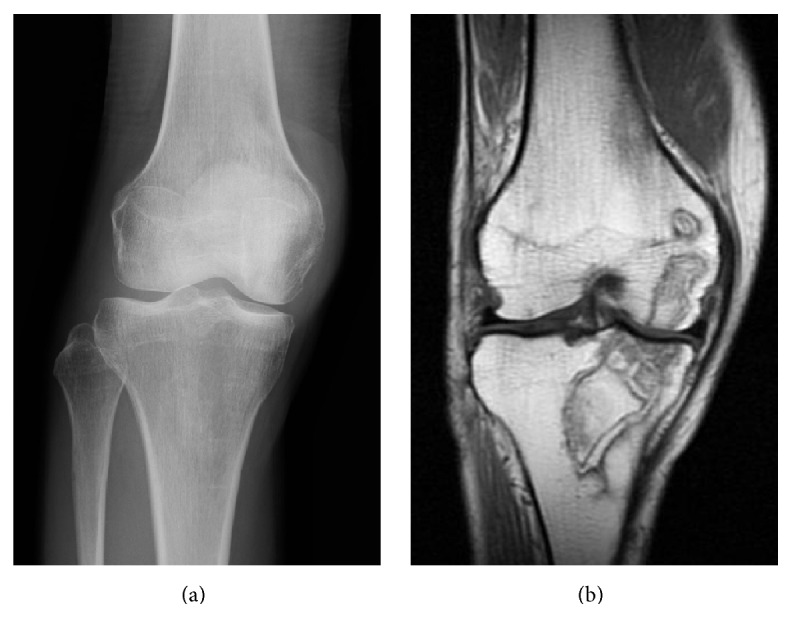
(a) Anteroposterior radiograph of the right knee showing increased radiodensity of the proximal half of the tibia. (b) T1W coronal section MRI showing large demarcated regions of abnormal signal intensity in the proximal half of the tibia and medial femoral condyle, with features suggestive of osteonecrosis.
